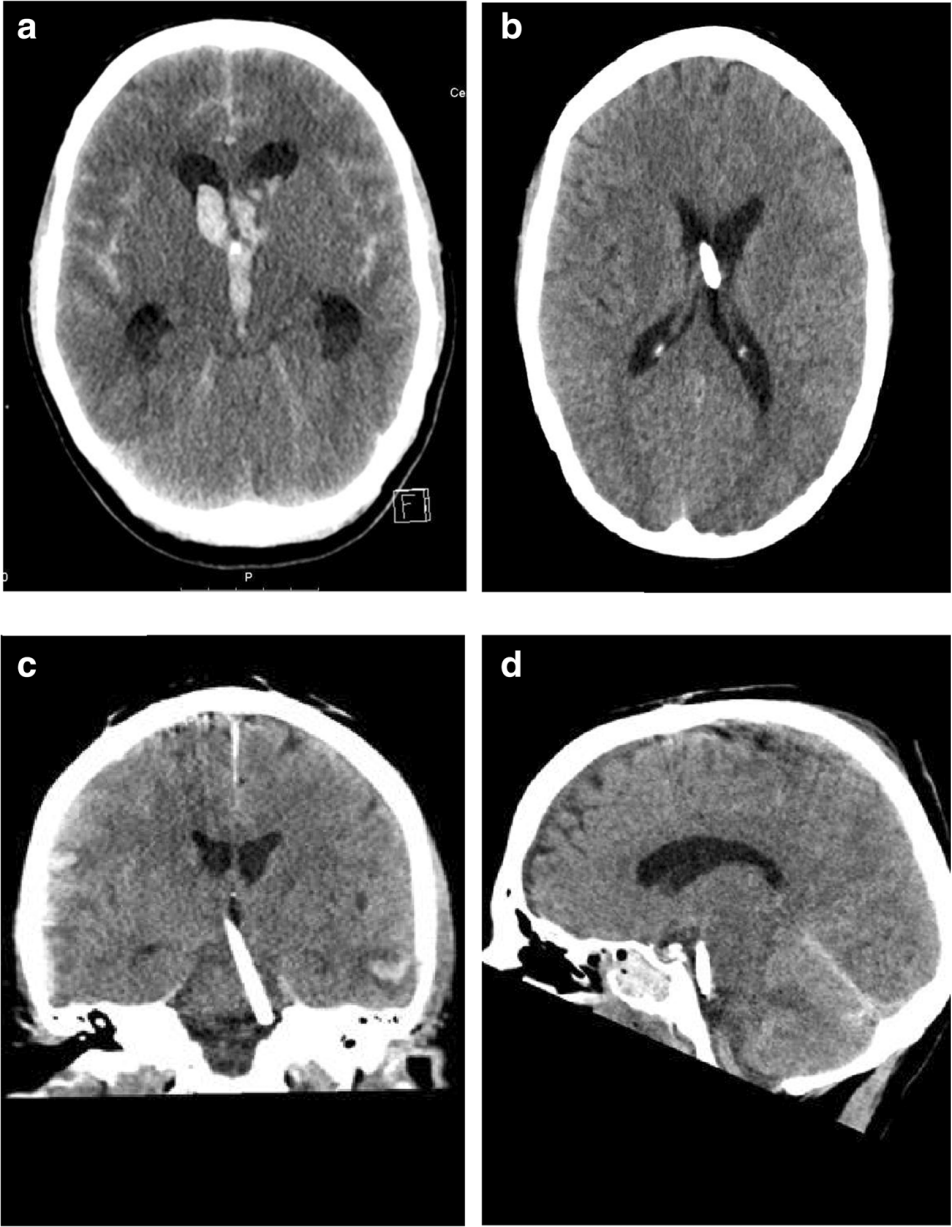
a–d Postoperative CT scans. a Kakarla score 1; tip of EVD in third ventricle. b Kakarla 2; tip of EVD in contralateral ventricle. c, d Kakarla 3; tip of EVD in basal cisterns-prepontine cistern (this patient underwent revision surgery)
